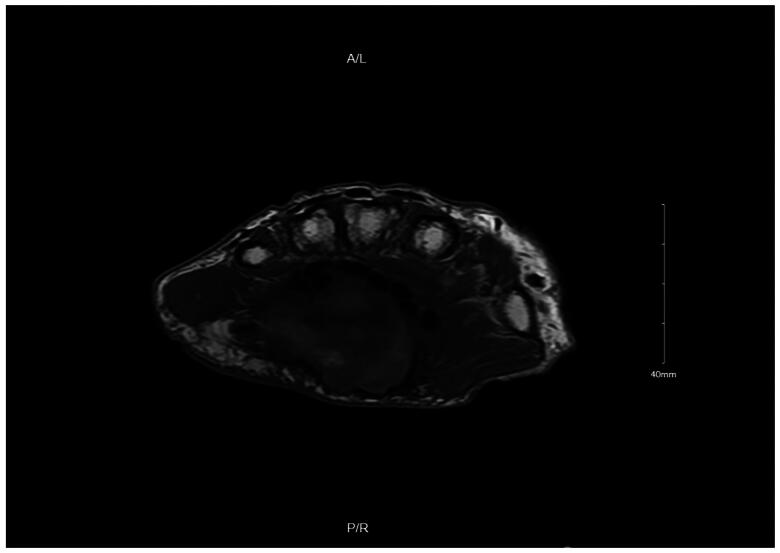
Coronal MRI of the left hand.
